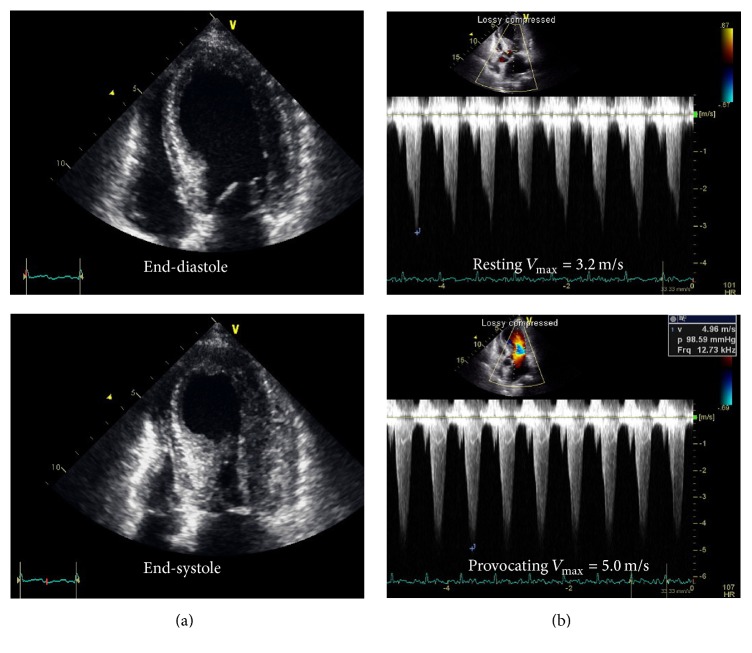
Transthoracic echocardiography showed an apical hypokinesia with normal left ventricular systolic function (a). Left ventricular outflow tract obstruction was confirmed by color flow acceleration with high-pressure gradient; maximal velocity (*V*_max_) 3.2 m/s at resting and 5.0 m/s after Valsalva maneuver (b).
